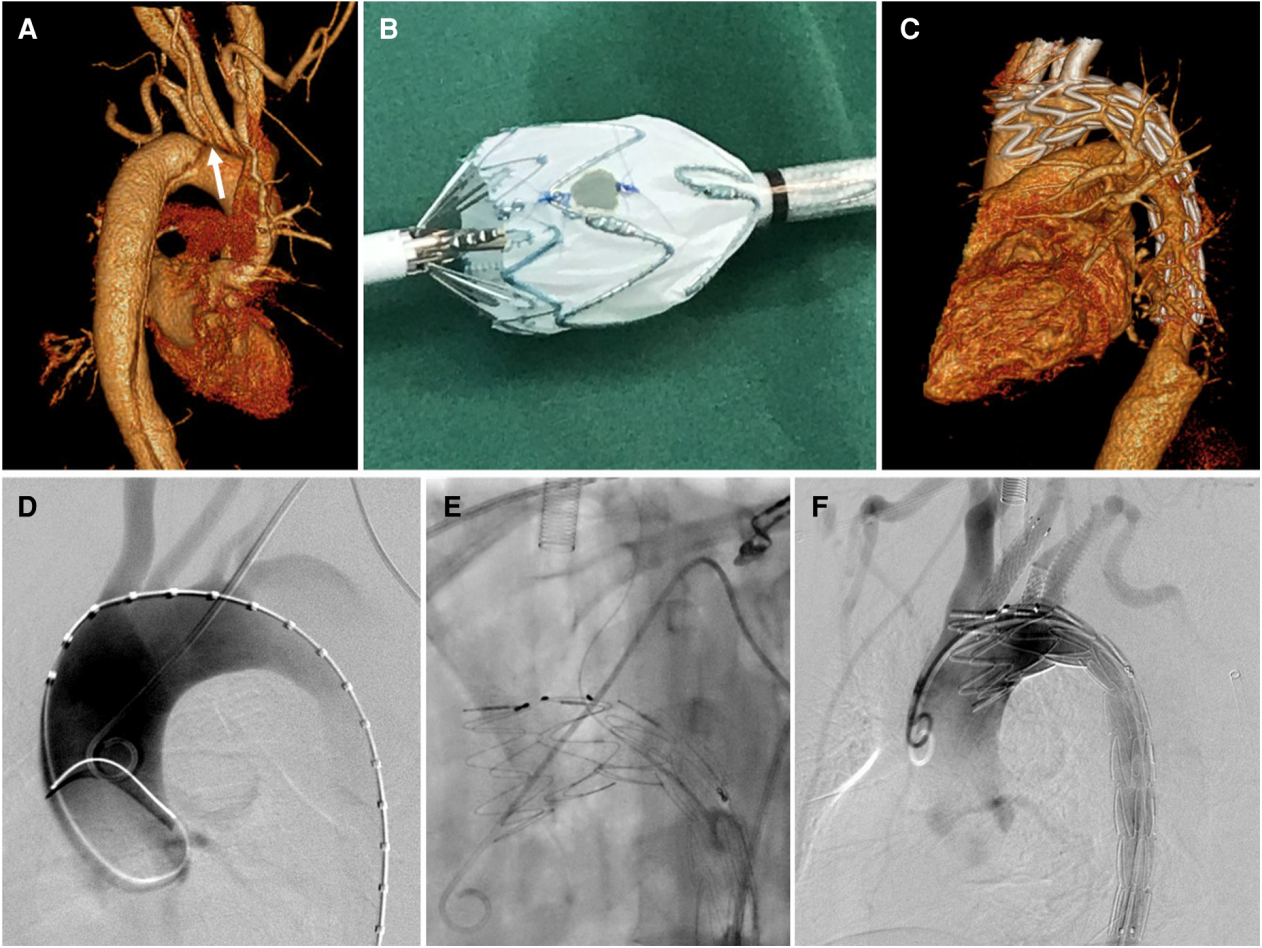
Physician modified-fenestration for isolated left vertebral artery. (A) Preoperative CTA of a type B aortic dissection demonstrating the ILVA (white arrow) from the distal aortic arch between the LCCA and the LSA; (B) A fenestration was made on the main body stent graft for preservation of ILVA on table; (C) follow up CTA showed patent target vessels and well excluded aortic dissection without endoleak; (D) intraoperative aortography showed a type B aortic dissection with a ILVA; (E) After full deployment of the stent graft, the ILVA was super-selected from the contralateral femoral access followed by bridging stent implantation; (F) Final aortography demonstrated complete exclusion of aortic dissection and patent aortic arch branch arteries, with bridging stent-grafts in the LCCA, ILVA, and LSA.
